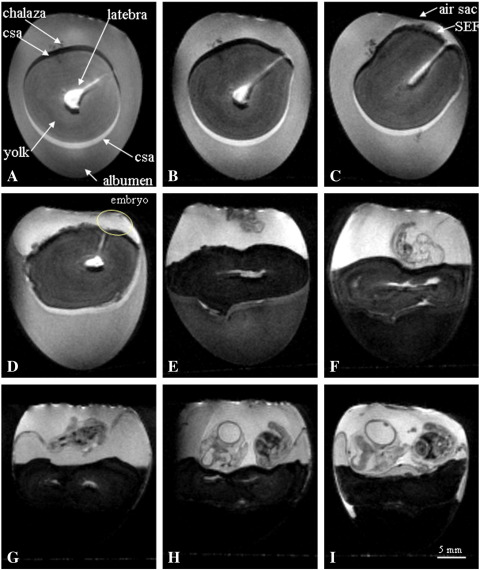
Two-dimensional RARE-8 vertical MR images from a 3D data set of a quail egg acquired longitudinally during the first 8 days of incubation. (A) Day 0; (B) Day 1; (C) Day 2; (D) Day 3; (E) Day 4; (F) Day 5; (G) Day 6; (H) Day 7; (I) Day 8. csa=Chemical shift artifact.
